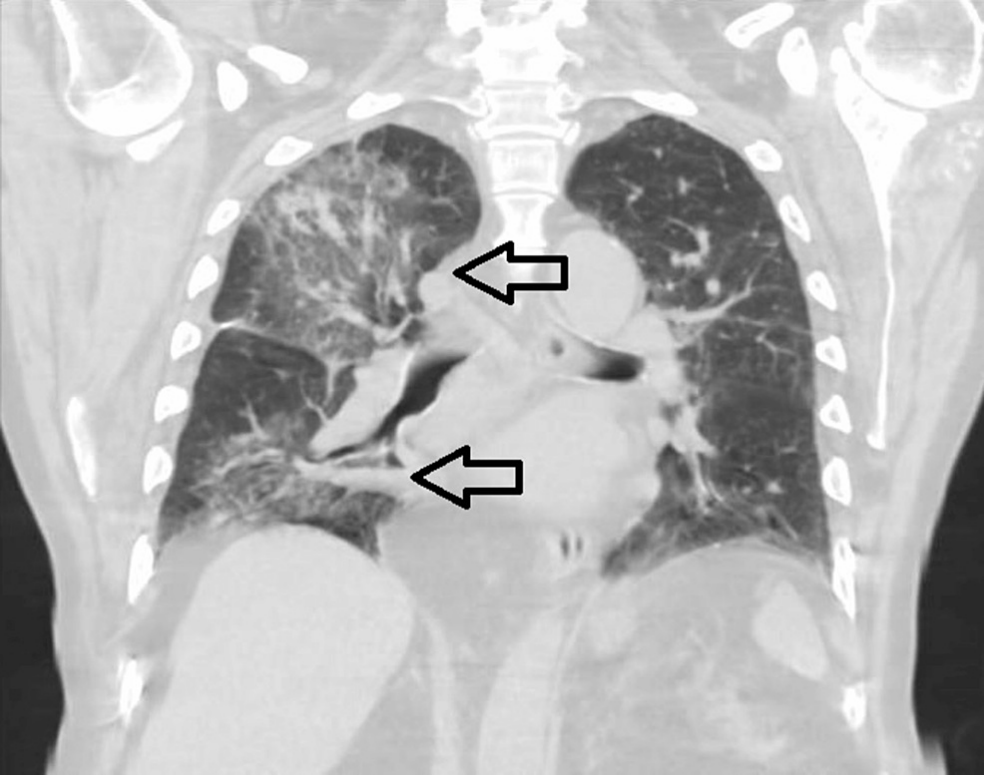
Computed tomography of the chest, coronal view, showing right-sided multi-lobar infiltrates
